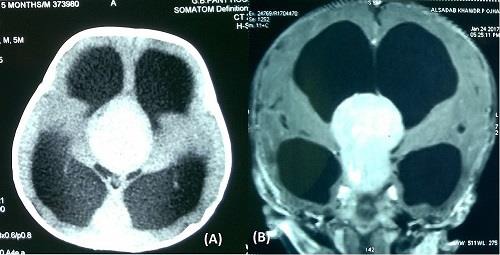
figures, computed tomography (A) and contrast T1-weighted magnetic resonance image (B) of a 4 months old child showing a tumor in suprachiasmatic region extending into third ventricle, which is homogeneously contrast enhancing. These radi-ological features are suggestive of optico-chiasmatic glioma.
